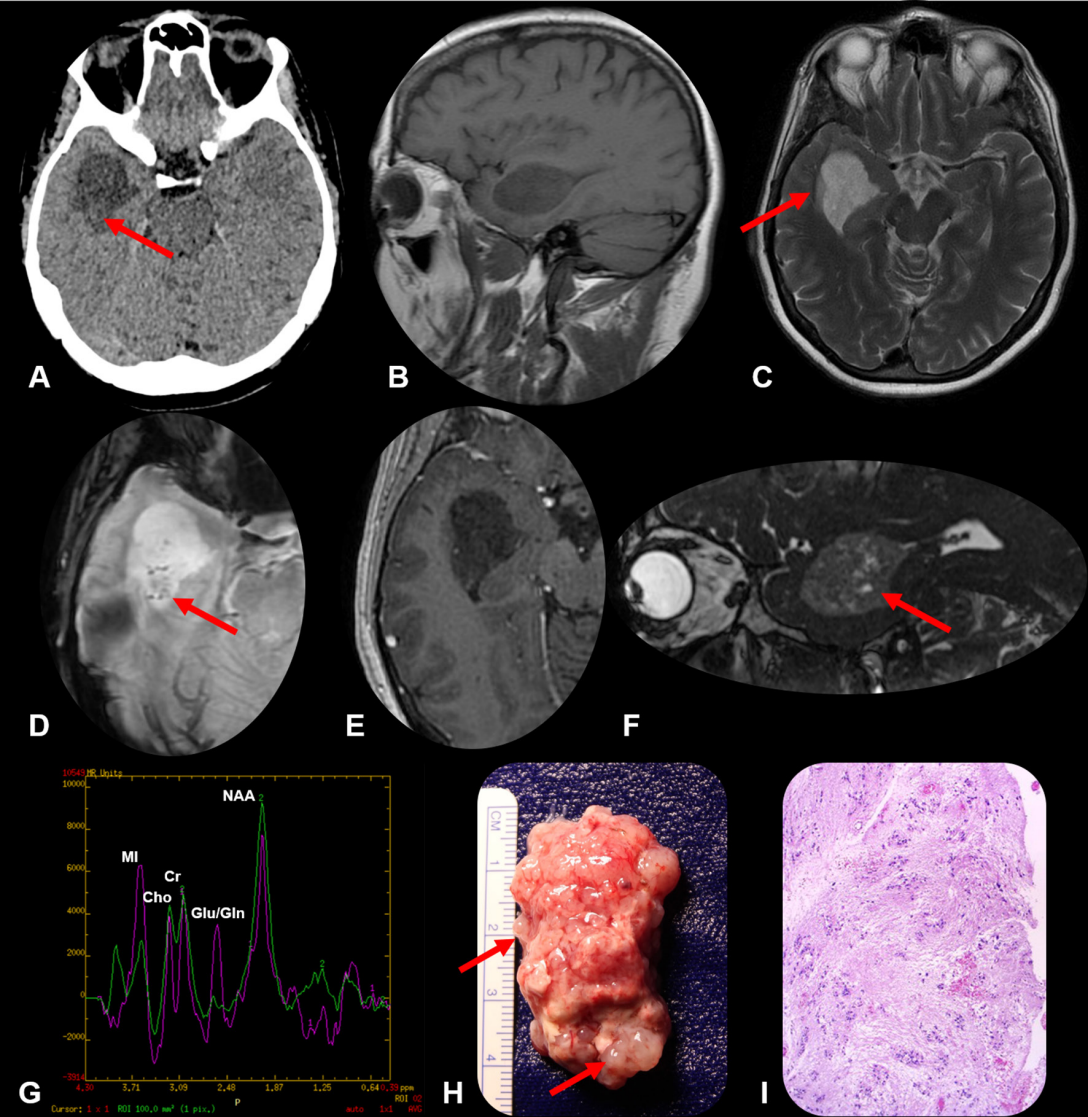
Non-contrast CT axial image shows a predominantly hypodense mass in the temporal horn of the right ventricle. Note there are tiny hyperdense foci (arrow), probably related to previous microhemorrhages (A) Sagittal *T*
